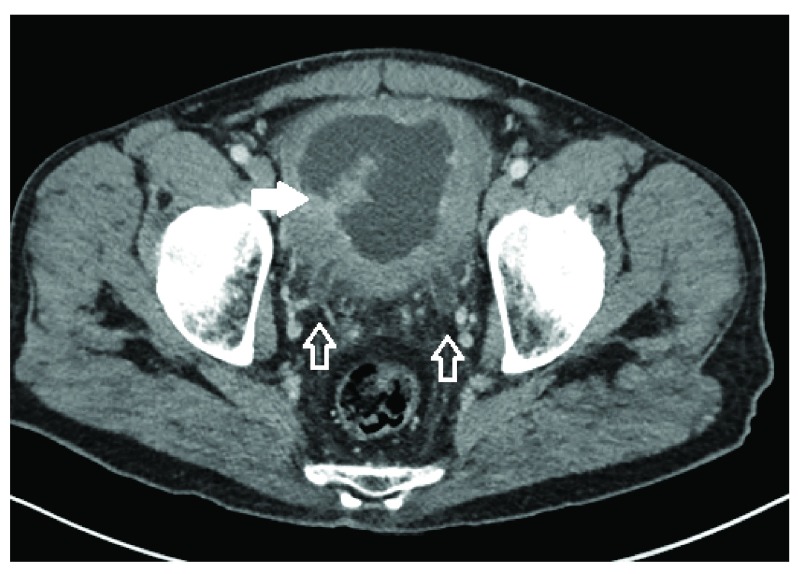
Axial contrast enhanced CT demonstrating bilateral ureteric dilation (hollow arrows) and a large enhancing intravesical mass (solid arrow).
